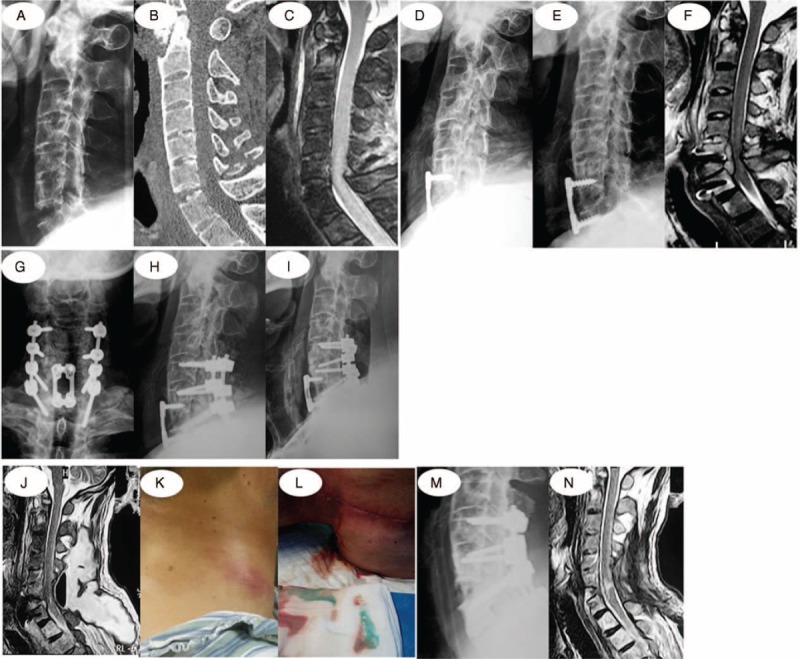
Main symptoms of a 49-year-old male patient suffering from cervical spine hyperextension injury caused by falling on ground with numbness and weakness. (A–C) X-ray and CT examination results show synostosis in each section of cervical spine with fracture separation at C6/C7, which belongs to I type, whereas MRI displays compression and edema in spinal cord. (D) In 2 segments fixed and fused with anterior approach, titanium plates and screws are well located at the end of the operation. (E, F) Five months after the operation, the patient expressed progressive neurological deterioration accompanied with internal fixation having slight dislocation shown by x-ray; besides, MRI examination reveals edema in prevertebral soft tissues, abnormal signals in C6/C7, high signals occupying posterior vertebral body, and spinal cord compressed. (G, H) Patient's neurological function was satisfactorily restored after posterior revision surgery. (I–L) Four months after revision operation when the patient developed fever coupled with mass in his right neck. X-ray and MRI examinations show swollen prevertebral soft tissues and gas in abscess cavity behind the neck, and these syndromes were confirmed as esophagus leakage by green dyestuff-like fester mixed in abscess cavity behind the neck after oral MeiLan. (M, N) Any leakage of contrast media could not be found after the oral omnipaque after 12 weeks of the following treatments: removing anterior cervical titanium plates and screws in second surgery, incision debridement, gastric intubation, enteral nutrition, and incision open drainage; in addition, abscess cavity, pneumatosis, or obvious abnormal signal shadow of soft tissues was not observed through MRI. All these indicate that esophagus leakage was healed. CT = computed tomography, MRI = magnetic resonance imaging.
